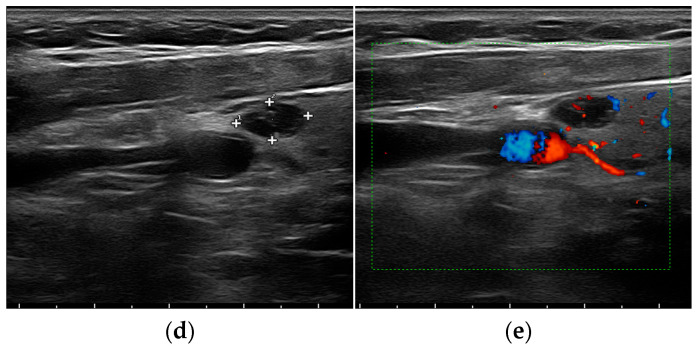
Grey-scale (a,b,d) and Color Doppler (c,e) aspect of a parathyroid adenoma.
